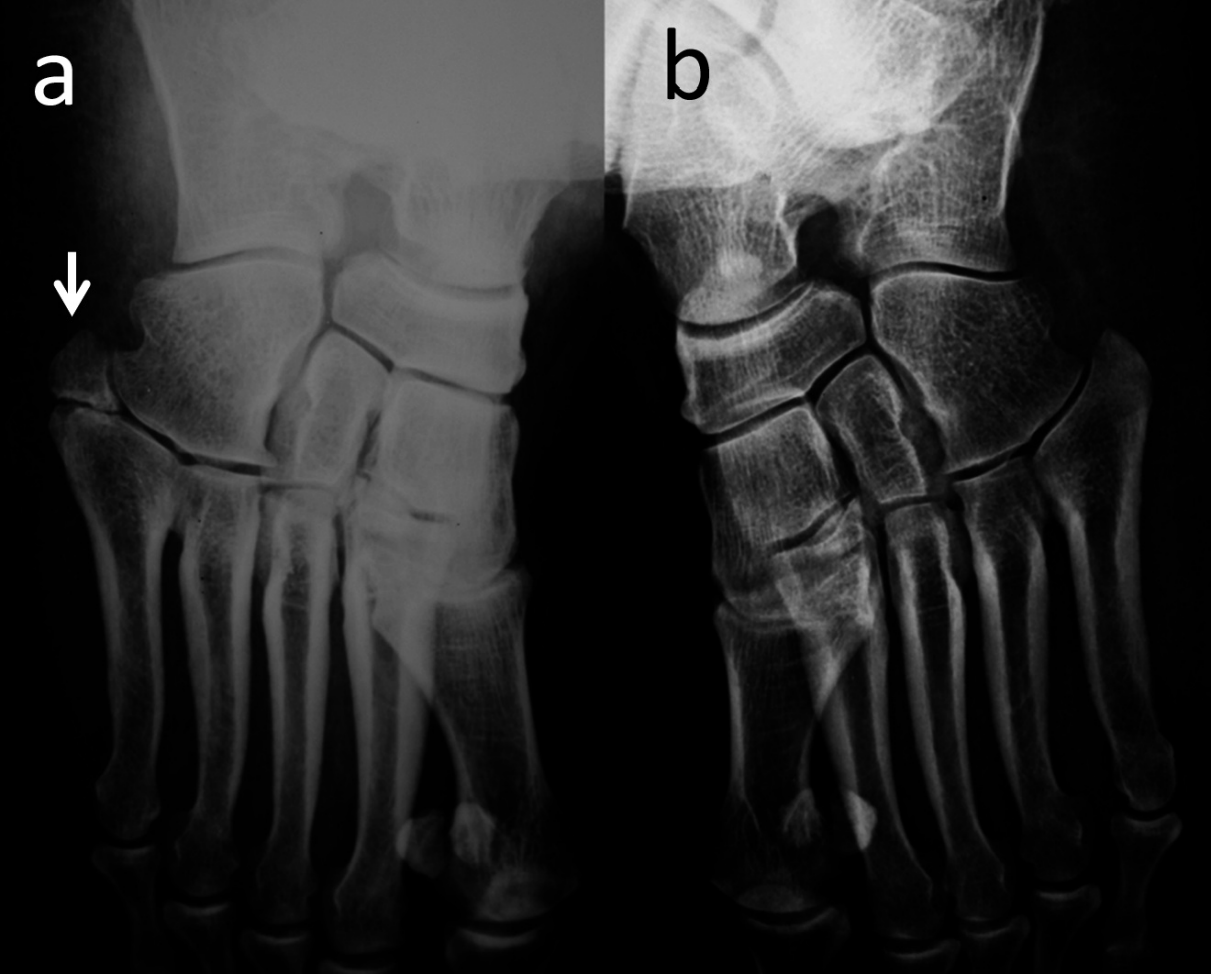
Bilateral anteroposterior foot radiograph of a patient with unilateral os vesalianum pedis (a) Right foot (white arrow) is os vesalianum pedis; (b) Normal left foot.
